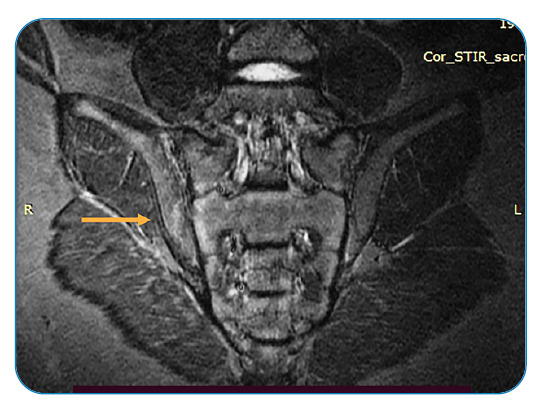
MRI showing effusion at the level of the right sacroiliac joint.
